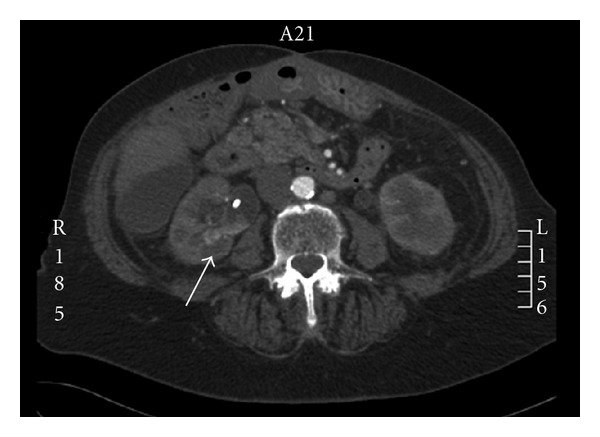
Computed tomography angiography (CTA) axial image; suspected active hemorrhage with clots in the right pyelocaliceal system.
